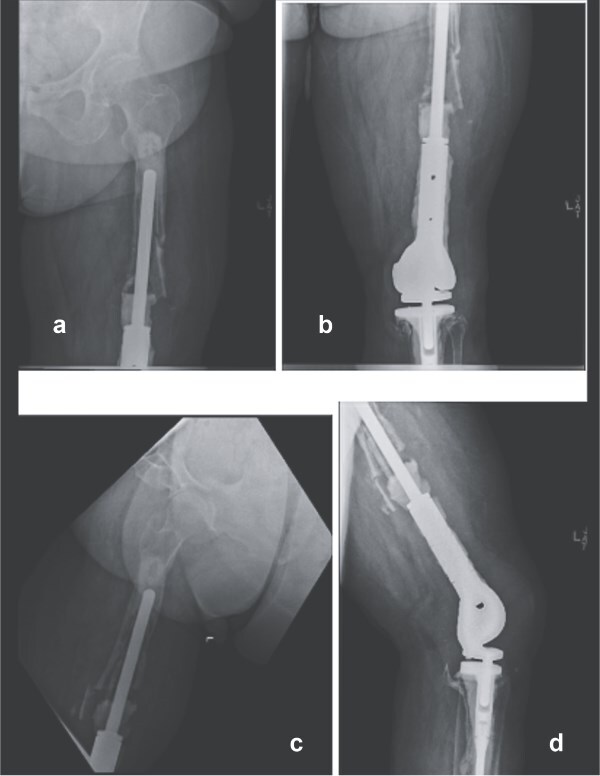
Preoperative anteroposterior (a and b) and lateral radiographs (c and d) of the right femur showing loosening of the proximal femoral stem and extensive proximal femoral bone loss following multiple distal femoral replacement revisions for osteosarcoma.
